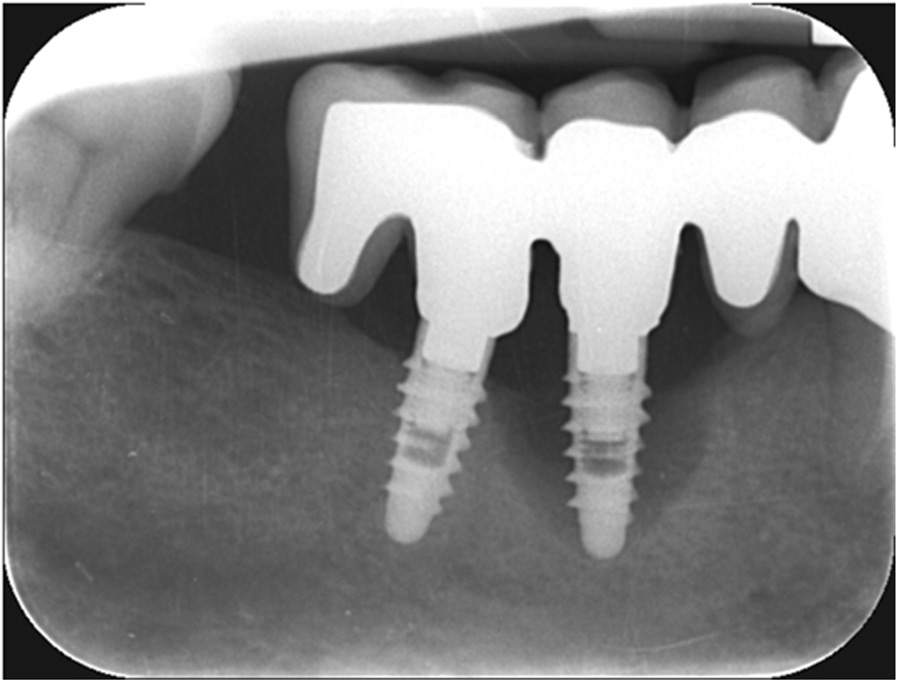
Intraoral radiography: the peri-implant defect
